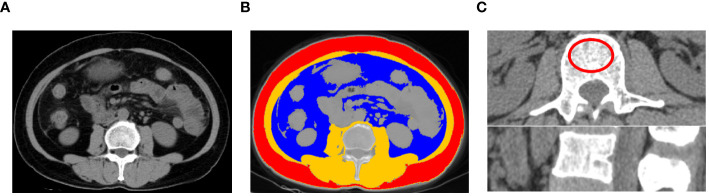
An example of segmentation of body composition. (A) original image; (B) Subcutaneous adipose tissue (red), visceral adipose tissue (blue), and skeletal muscle area (Brown) from an axial image at the level of L3 vertebra of a CT scan; (C) Measurement of bone mineral density of L2 vertebra a CT scan.
